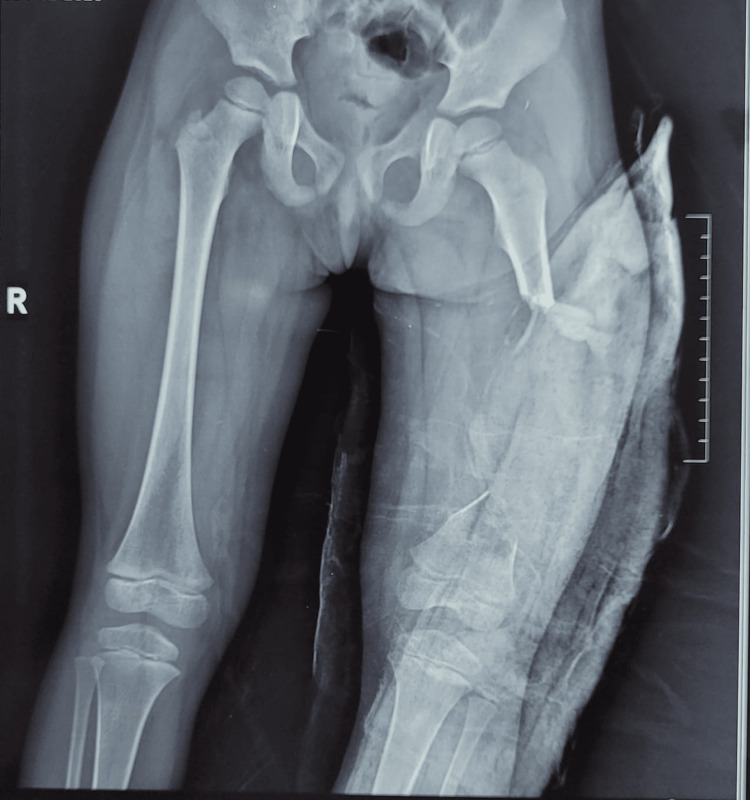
Anteroposterior (AP) view radiograph showing missing segment of the left femur (the limb was immobilised in a high groin plaster of Paris slab).
